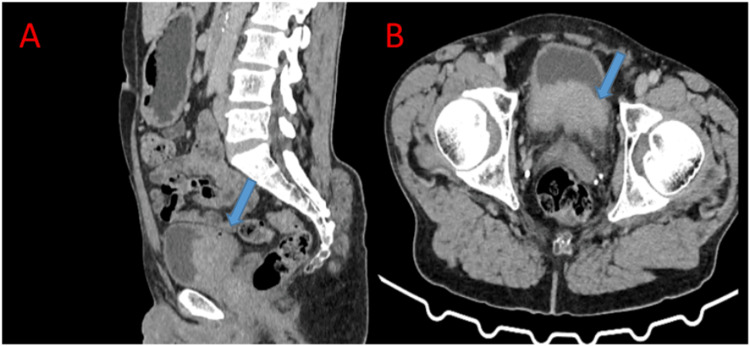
CT urography demonstrates infiltrative bladder wall thickening involving the ureteral orifices and the bladder base, with extension into the perivesical fat A: sagittal section; B: axial section.
